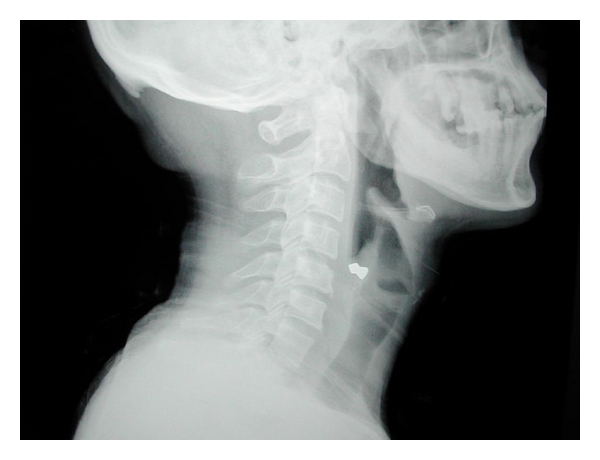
X-ray lateral view showing a radio-opaque foreign body at the junction of C5-C6 vertebrae (level of laryngeal ventricle).
